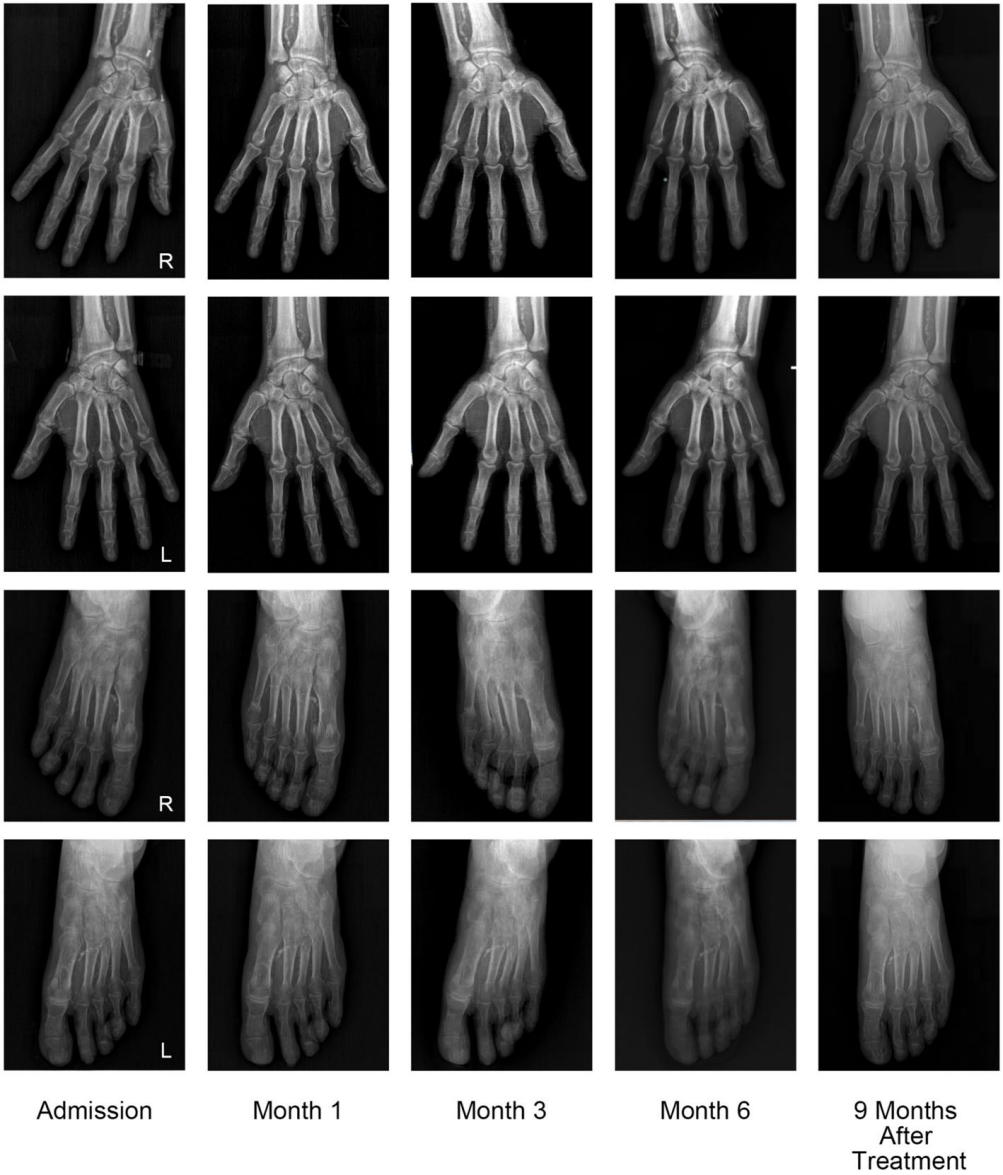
Plain radiographs of the hands and feet show severe vascular calcification and no significant reversal after treatment
